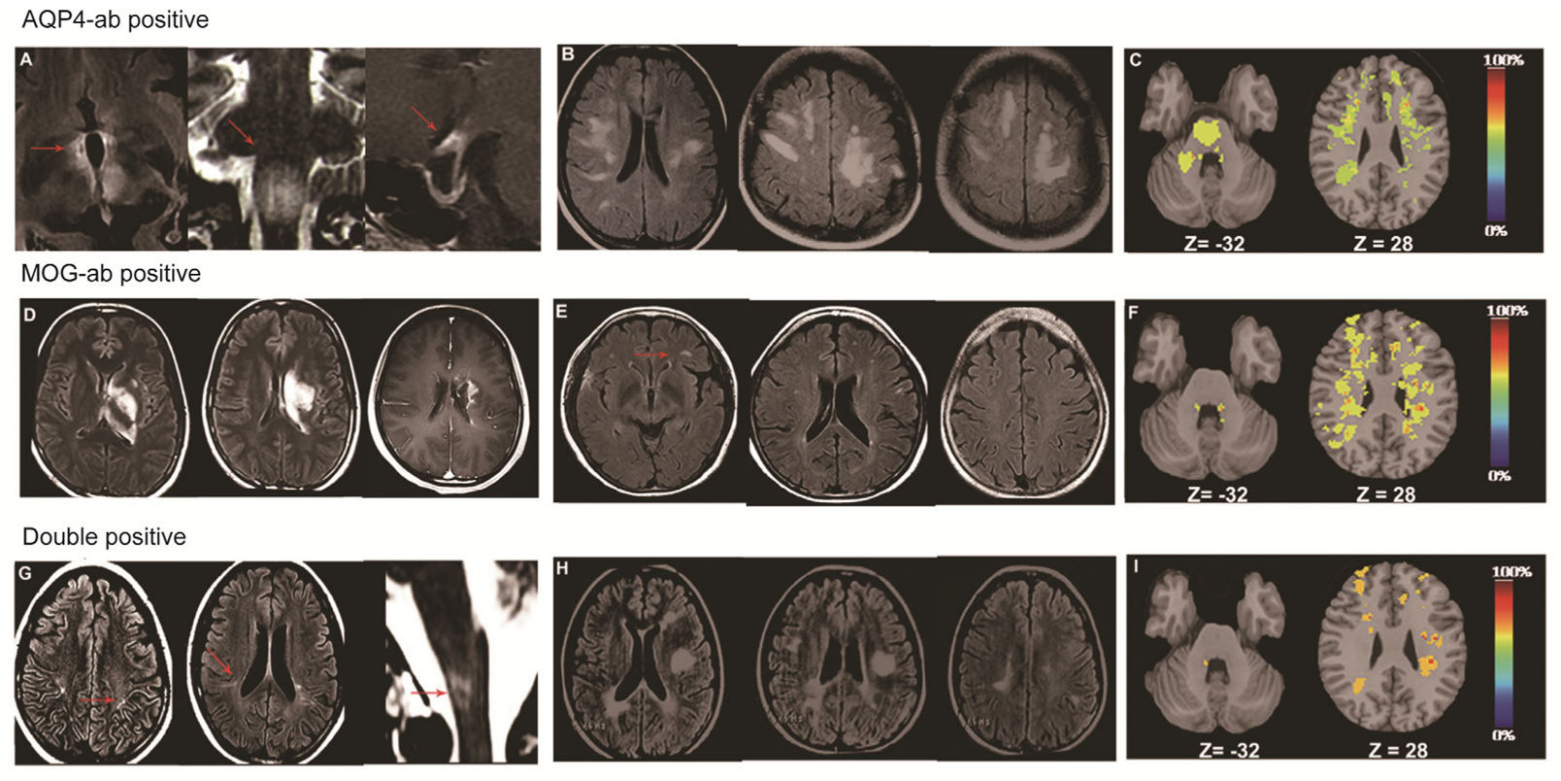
Brain lesions in MRIs of NMOSD patients with different antibody profiles during attacks. A–C, NMOSD patients with AQP4-ab. D–F, NMOSD patients with MOG-ab. G–I, NMOSD patients with both AQP4 and MOG-abs. A, Typical NMO-like lesion: fluid-attenuated inversion recovery sequence (FLAIR) with T2-weighted hyper-intensities evident in peri-ependymal areas and the diencephalon, including bilateral hypothalamic lesions (A left, arrows); T2-weighted hyperintensities in the floor of the fourth ventricle, bilateral area postrema lesion (A middle, arrow); chiasmal lesion with gadolinium enhancement (A right, arrow). B, ADEM-like lesion without enhancement: patient with extensive bihemispheric subcortical white matter FLAIR signals abnormality without enhancement. The lack of enhancement suggests an intact blood-brain barrier and supports a unique mechanism of edema induction from the dysfunction of water channels. C, Lesion probability distribution for NMOSD patients with AQP4-ab (*n*=26): the color scale (from 0% to 100%) represents the minimum to maximum probability of a lesion occurring in a particular spatial location. Montreal Neurological Institute (MNI) standard space template Z coordinate is shown in millimeters. D, AMED like lesions with enhancement: a patient with MOG-ab had a large confluent FLAIR signal abnormality in the left putaman, thalamus and paraventricle white matter that demonstrates diffuse gadolinium enhancement. Emerging enhancement suggests disruption of the blood-brain barrier and supports an inflammatory mechanism producing edema. E, MS-like lesion but lesions were too few to satisfy the Barkhof criteria for MS (arrow). F, Lesion probability distribution for NMOSD patients positive for MOG-ab (*n*=8). G, Small MS-like lesions satisfy Barkhof criteria, also a special NMO-like lesion with area postrema lesion surprisingly happened in the same patient (arrow). H, Large typical MS-like lesions satisfy Barkhof criteria. I, Lesion probability distribution for NMOSD patients with MOG-ab and AQP4-ab (*n*=10).
